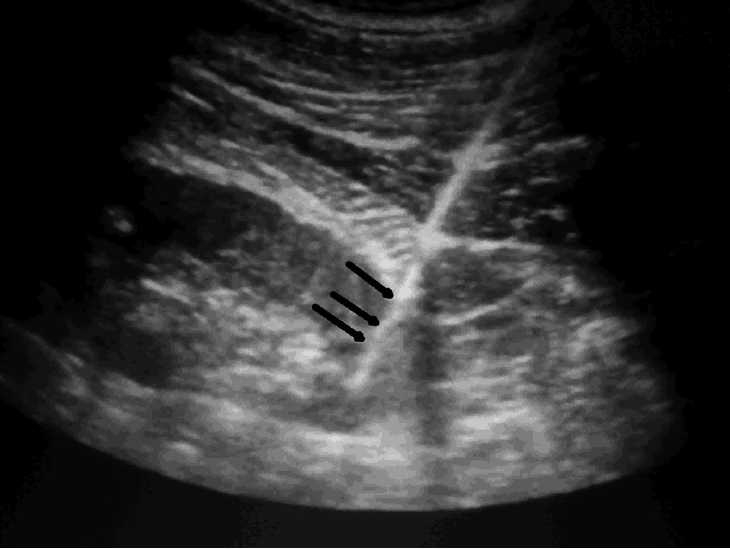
Ultrasound image showing the biopsy gun inside the lower pole of the kidney (arrows)
